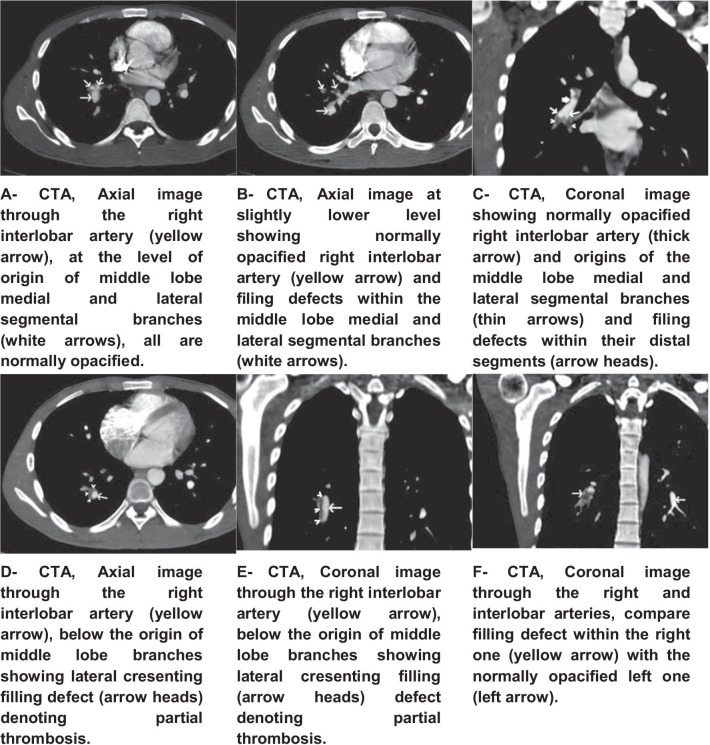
Spiral chest CT scan angiography
